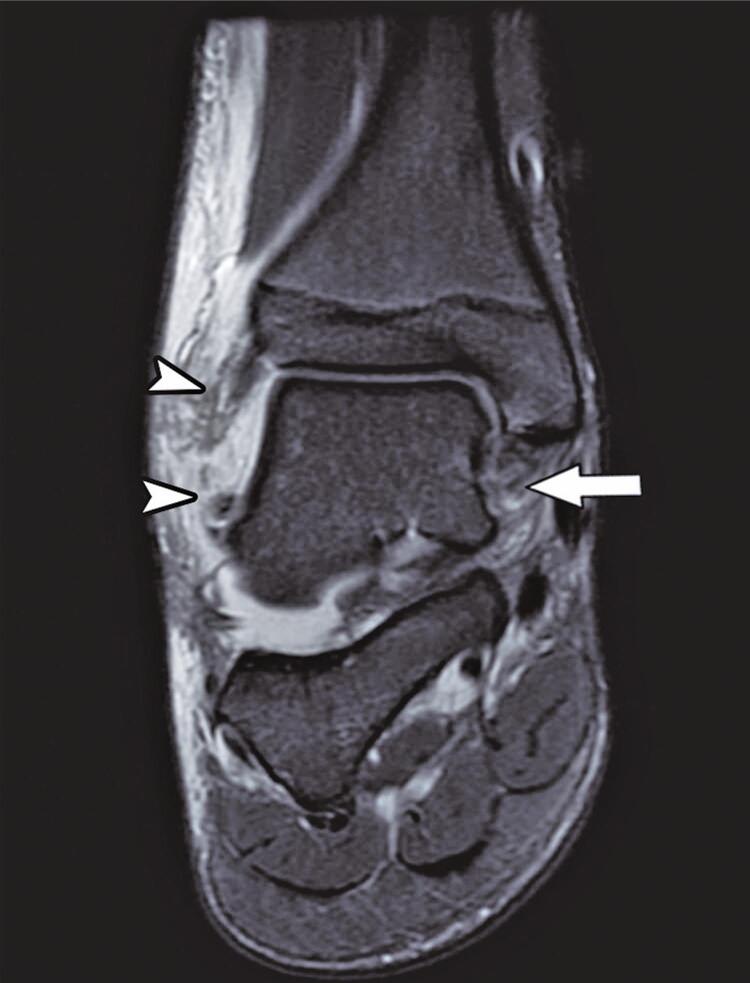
Coronal T2-weighted fat-suppressed magnetic resonance image presents partial tear of the deltoid ligament (arrow) and complete tear of the lateral ligament complex (arrowheads, partially seen on this image). Extensive lateral ankle soft tissue edema and subtalar joint effusion are also depicted
